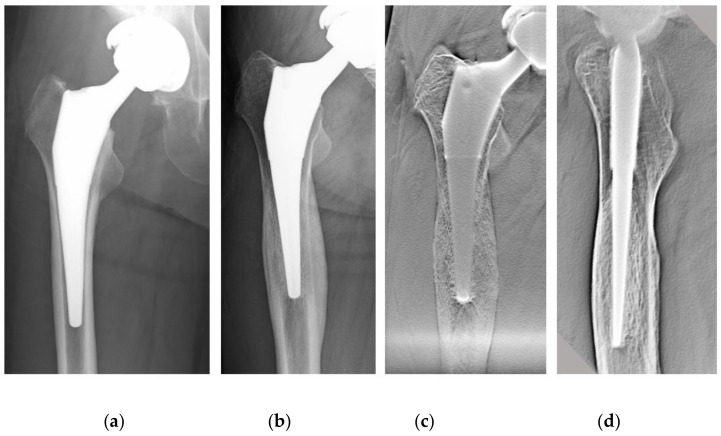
A 61-year-old female patient after THA using a tapered wedge stem. (a) Plain radiographs one month after THA. (b) The same patient 6 y later. There was cortical hypertrophy at Gruen Zones 3 and 5. (c) DTS (AP view) at the same time as (b). Cortical hypertrophy was observed more clearly. (d) DTS (lateral view) at the same time as (b). Heterogeneous loss of permeability is seen in the cancellous bone distal to the porous coating. THA, total hip arthroplasty; DTS, digital tomosynthesis; AP, anterior–posterior.
